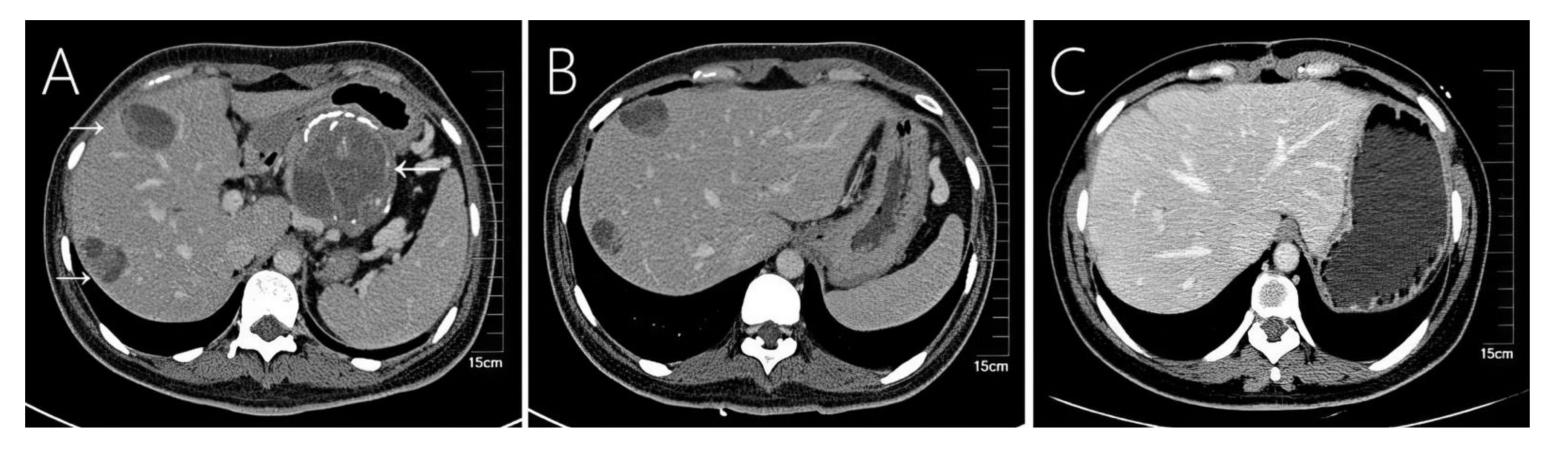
Case 2 shows a 36-year-old woman’s abdomen with contrast-enhanced CT scan showing a well-encapsulated heterogeneous mass in the tail of the pancreas. (A) The primary tumor showed eggshell-like calcification, and two liver metastases were seen in the liver, showing a mixed structure of cysts and solids. (B) Compared with preoperative CT at almost the same level, (C) the 54th month postoperative follow-up shows regular liver morphology and no other liver metastases.
